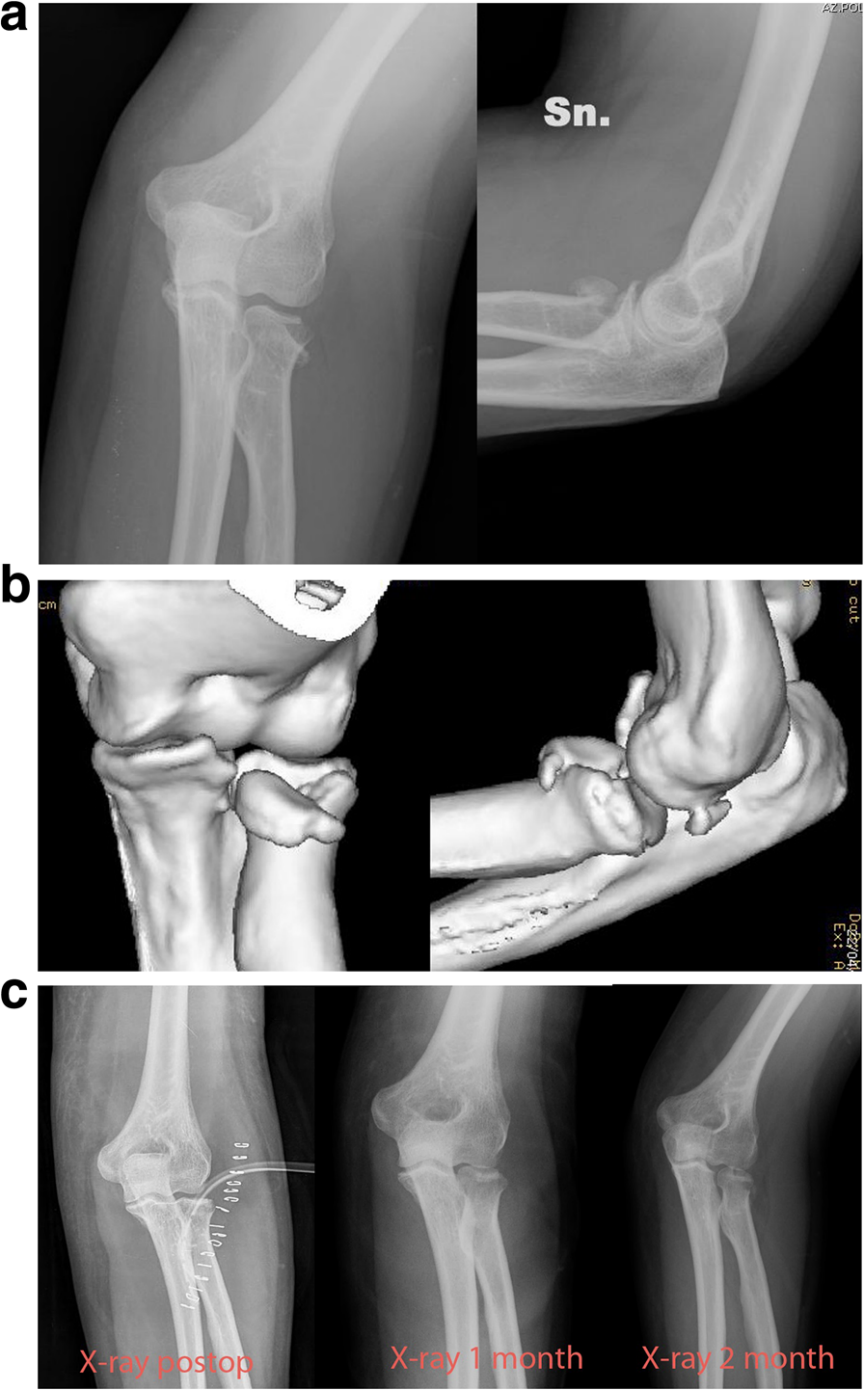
a Severely displaced Mason type II fracture. b 3D-computed tomography study. c X-rays follow-up with secondary displacement of fracture fragments of about 3 mm observed 2 months after surgery
